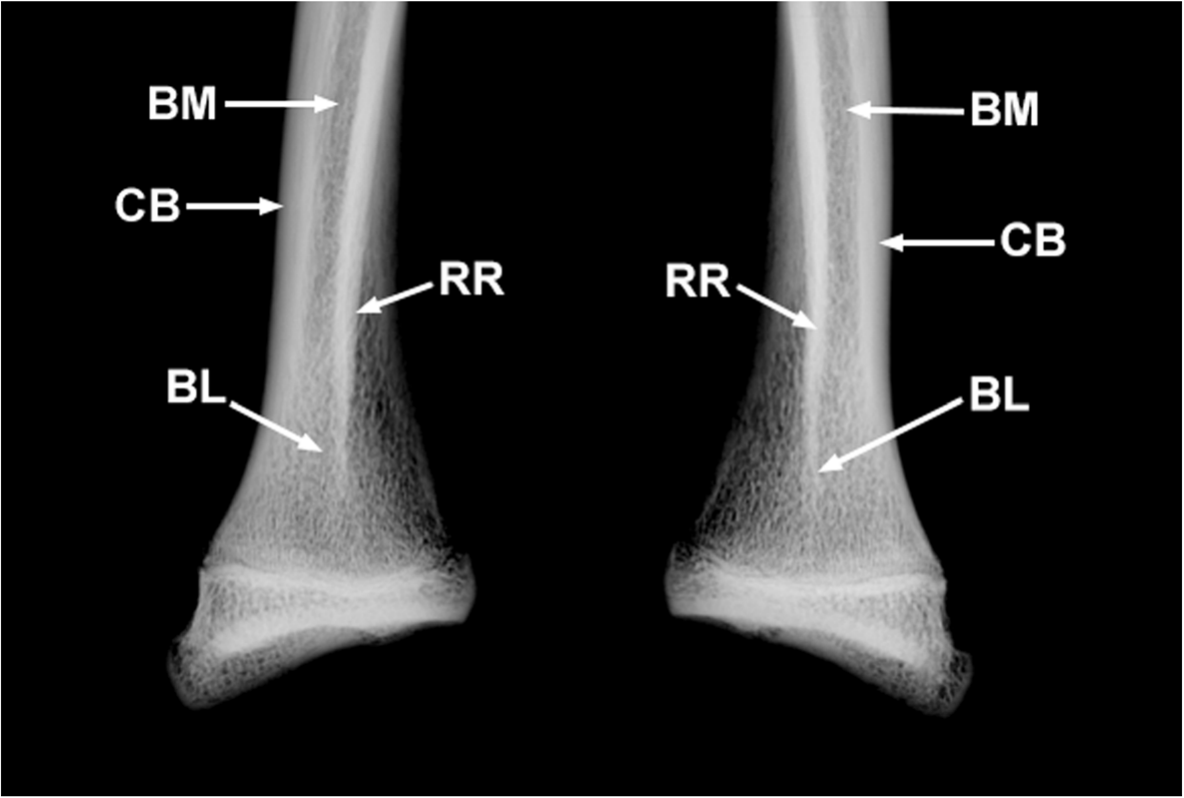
Radiographs of the injured distal third of the radii of *Tamandua tetradactyla* (MN 79028). BL, Bone loss; BM, Bone marrow; CB, Cortical bone; RR, Radial ridge
